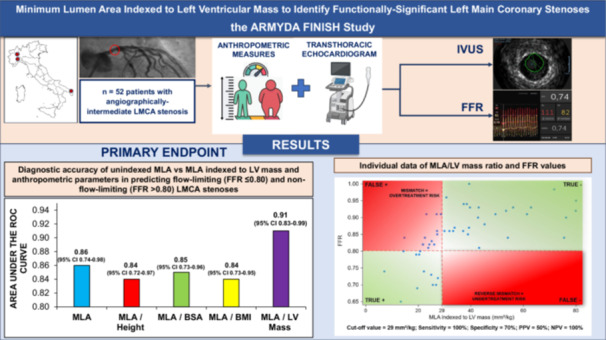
Study overview and main findings. In patients with angiographically‐intermediate LMCA disease, IVUS‐derived MLA indexed to echocardiographic LV mass achieved the highest area under the receiver operating characteristic curve (AUC = 0.91) in predicting flow‐limiting LMCA stenoses (FFR ≤ 0.80), compared to unindexed MLA (AUC = 0.86) and MLA indexed to height, BSA or BMI (AUC range = 0.84–0.85). At a cutoff of 29 mm^2^/kg for MLA/LV mass, sensitivity and NPV ranged both 100%, minimizing the risk of undertreatment. AUC = area under the curve, BMI = body mass index, BSA = body surface area, FFR = fractional flow reserve, IVUS = intravascular ultrasound, LMCA = left main coronary artery, LV = left ventricular, MLA = minimum lumen area, NPV = negative predictive value, PPV = positive predictive value, ROC = receiver‐operating characteristic. [Color figure can be viewed at wileyonlinelibrary.com]
